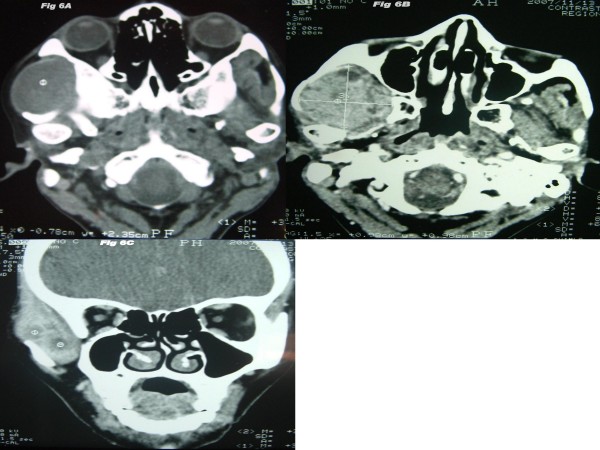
A-C: CT scan (axial section) showing hypodense (37 HU) mass in the right temporal and infratemporal region (Fig. 6A). The mass had heterogeneous enhancement (66 HU) in contrast CT scan (axial and coronal sections) (Fig 6B-C). The adjoining muscles are indistinguishable from the mass.
